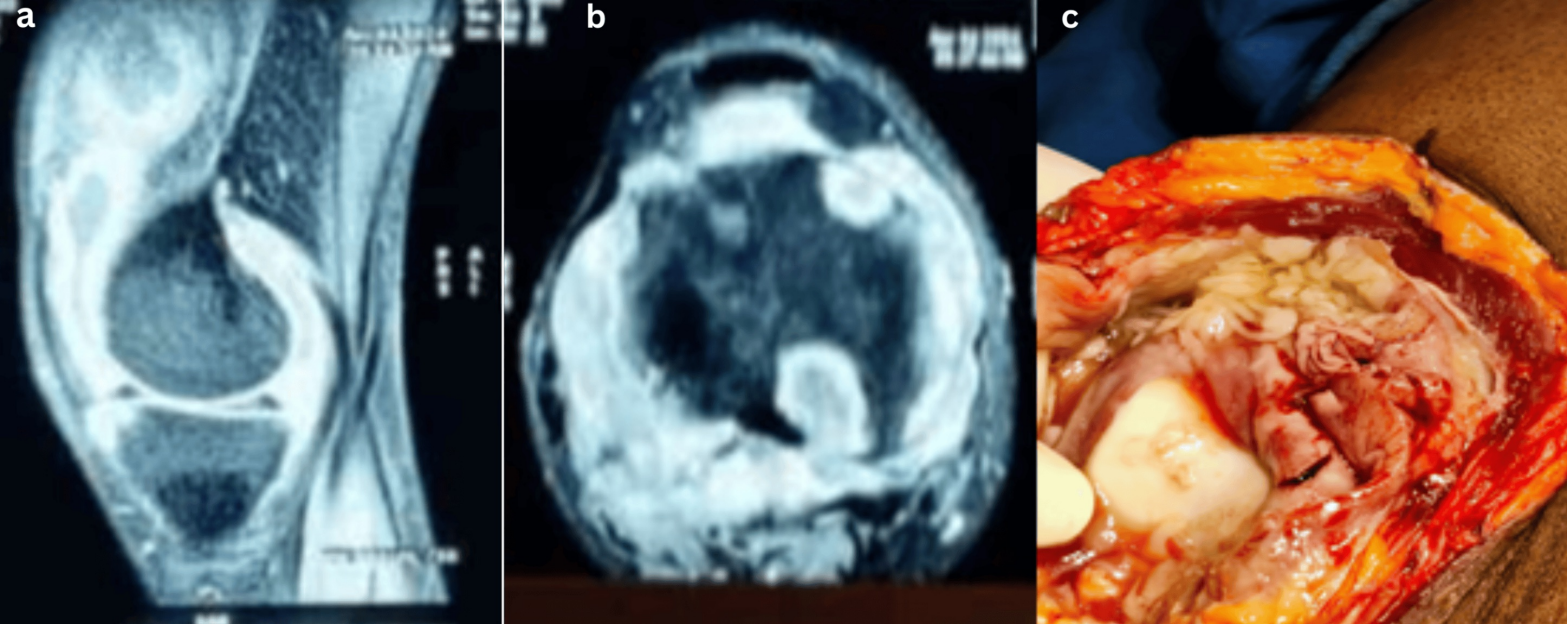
Extraspinal case 1: knee TB (a, b) MRI of the right knee joint in the sagittal and axial views, respectively, shows extensive synovitis with moderate knee joint effusion and multiple marginal erosions and patchy areas of bone marrow edema, suggestive of infective arthritis. (c) Intraoperative picture of the right knee joint showing caseous necrotic material within the joint cavity, surrounded by hypertrophied synovium with a thick, pale, and fibrillar appearance. The adjacent soft tissue and capsule appear hyperemic and edematous. Data shown are representative MRI and intraoperative findings from a single patient case (extraspinal case 1: knee TB). Original images obtained by the authors at Subbaiah Institute of Medical Sciences, Shivamogga.
